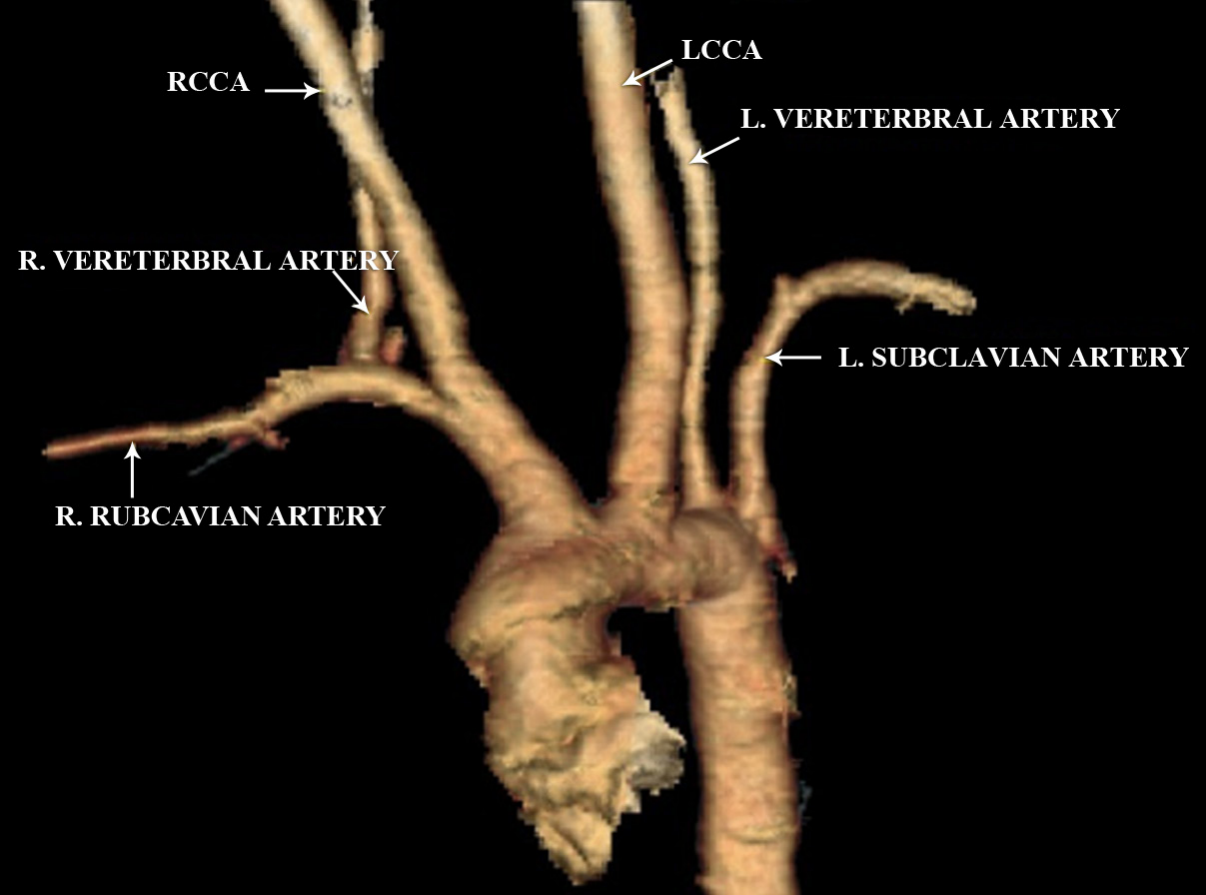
Three-dimensional computed tomography angiography scan of the aortic arch, coronal view, showing the abnormality of the aortic branches and left vertebral artery originating from the aortic arch.
